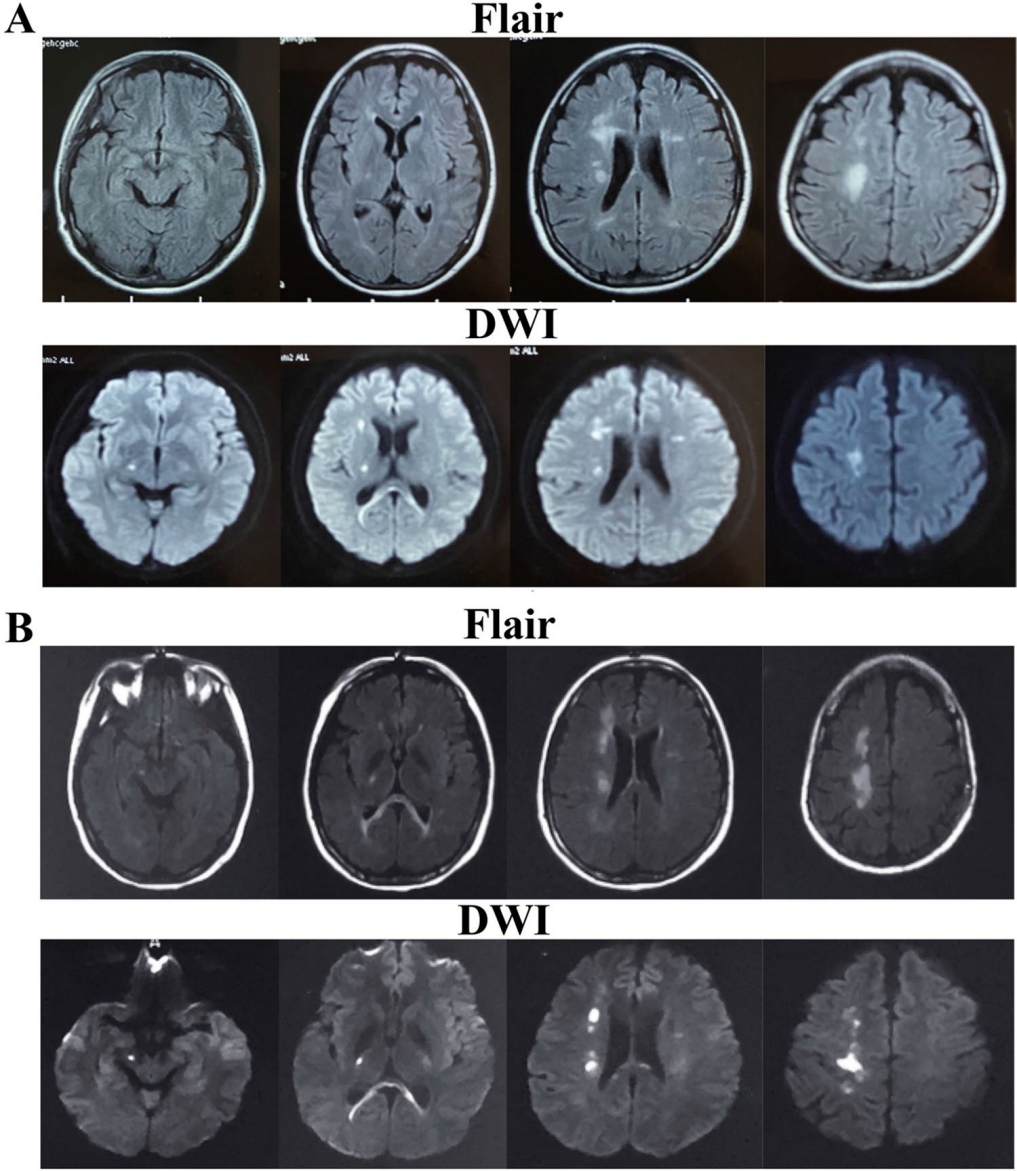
Magnetic resonance imaging (MRI) of the patient. A In March 2019, when the proband had no symptoms, the fluid-attenuated inversion recovery (FLAIR) and diffusion-weighted imaging (DWI) hyperintense signals were observed in the bilateral corona radiata, the splenium of corpus callosum, and the right basal ganglia region. B In October 2019, when the proband complained of the weakness of her left lower limb, MRI demonstrated more abnormal signals in the right corona radiata regions and the centrum semiovale than those in March 2019
